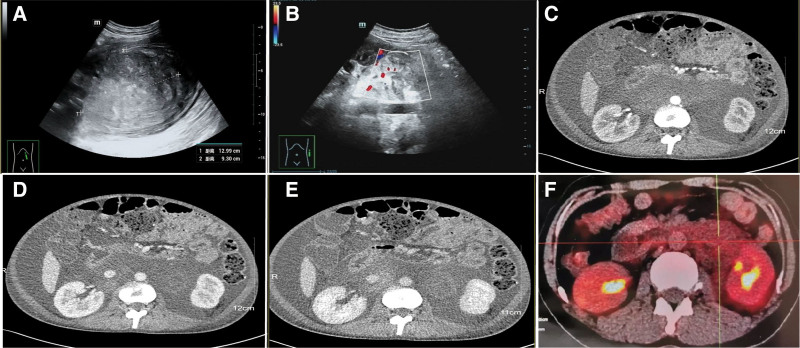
Imaging features of a 32-year-old woman with a retroperitoneal mass (A and B) Ultrasound revealed multiple hypoechoic nodules in the retroperitoneal area, with indistinct borders and an irregular echo pattern. CDFI showed minimal blood flow, suggesting a fibrotic nature of the mass. (C–E) CT scan demonstrated a retroperitoneal mass with a shadow. The 3-phase enhancement did not show any significant increase in intensity, indicating a nonmalignant lesion. (F) PET-CT showed patchy hyperdensity in the retroperitoneal space, with slightly increased FDG metabolism diffusely, suggesting inflammation or fibrosis rather than malignancy. Clinical significance: The imaging features, including ultrasound, CT, and PET-CT findings, assist in differentiating RPF from other retroperitoneal pathologies, such as malignancies. The absence of significant enhancement on CT and the lack of increased FDG uptake on PET-CT support a diagnosis of RPF rather than cancer. CDFI = Color Doppler flow imaging, CT = computer tomography, PET-CT = positron emission tomography-computed tomography, RPF = retroperitoneal fibrosis.
